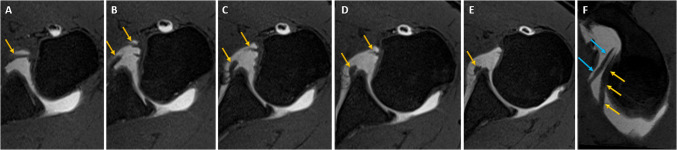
Consecutive axial A–E and oblique sagittal F thin-slice VIBE T1-weighted MR arthrograms reveal high-origin AB-IGHL (yellow arrows) inserting into 2 o’clock quadrant and normal MGHL (blue arrows)
